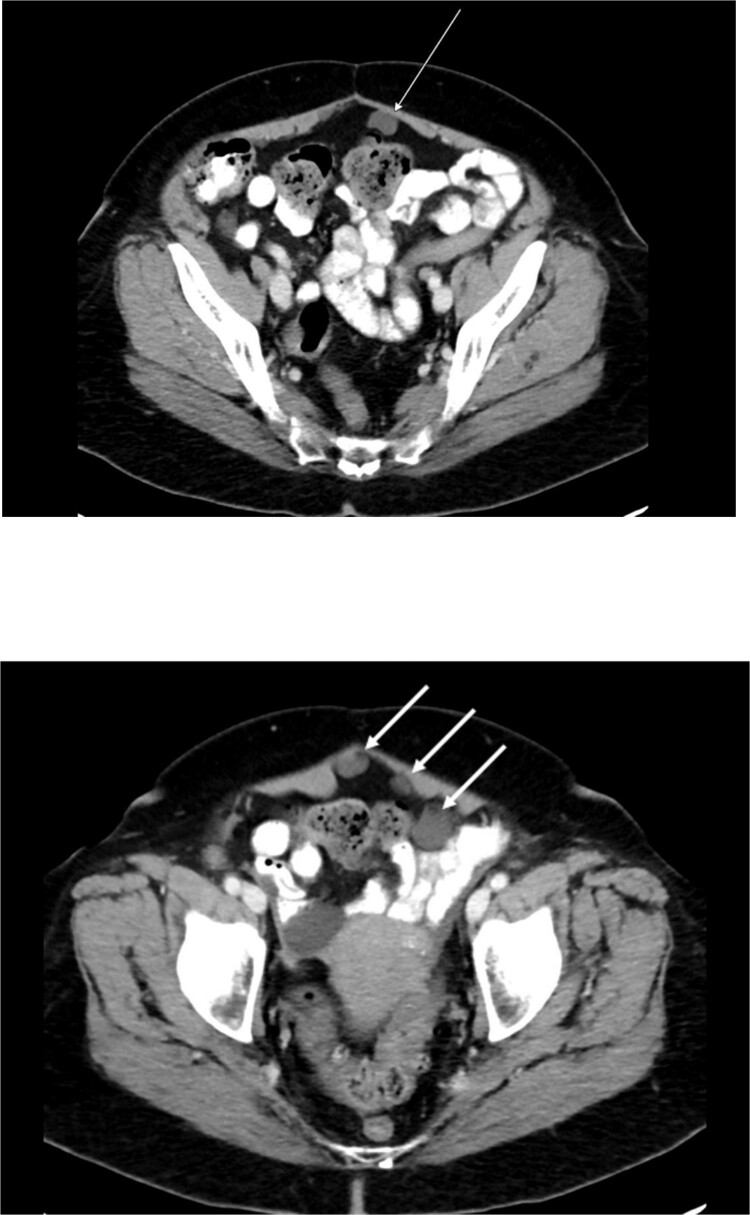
Computed tomography abdomen/pelvis—arrows indicate peritoneal cysts.
